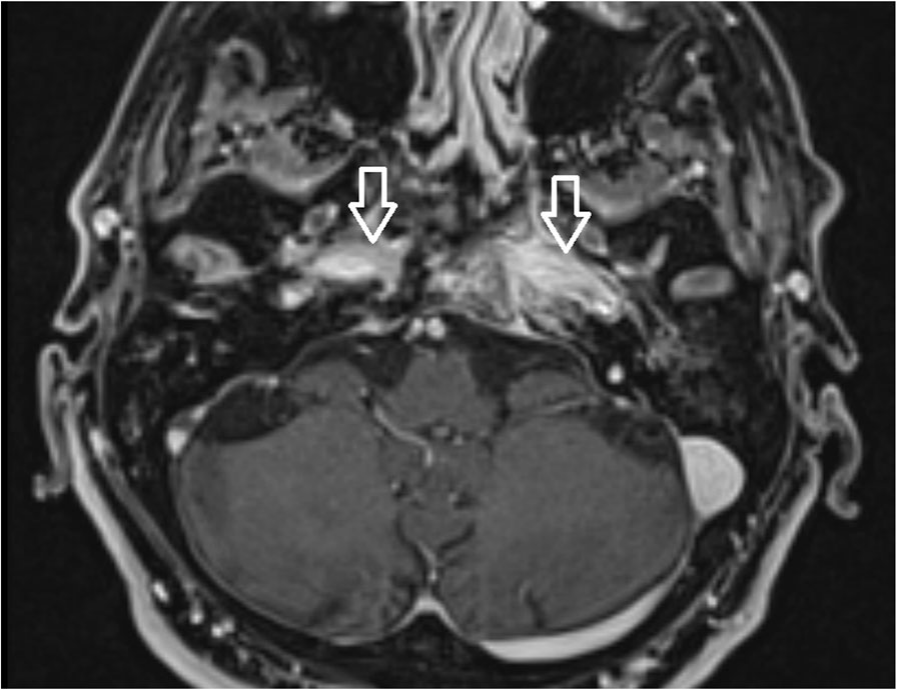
Gadolinium-enhanced axial T1-weighted spin-echo image with fat saturation. In advanced stages of an infection, a butterfly-shaped soft tissue enhancement was described underneath both petrosal bones and the clivus (arrow)
